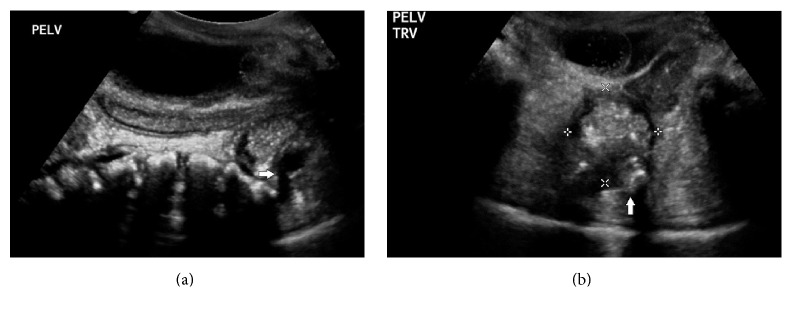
Abdominal ultrasound on day 7 to evaluate the neuroenteric fistula. (a) Gray scale sagittal image demonstrates a complex presacral mass with heterogeneous echogenicity and multiple echogenic foci representing air. There is a hypoechoic cystic structure within it which is in continuity with the sacral canal (arrow). (b) Subsequent transverse gray scale US image shows echogenic foci representing air in the small inferior hypoechoic cyst with the presacral mass (within calipers) suggesting continuous fistulous connection (arrow).
